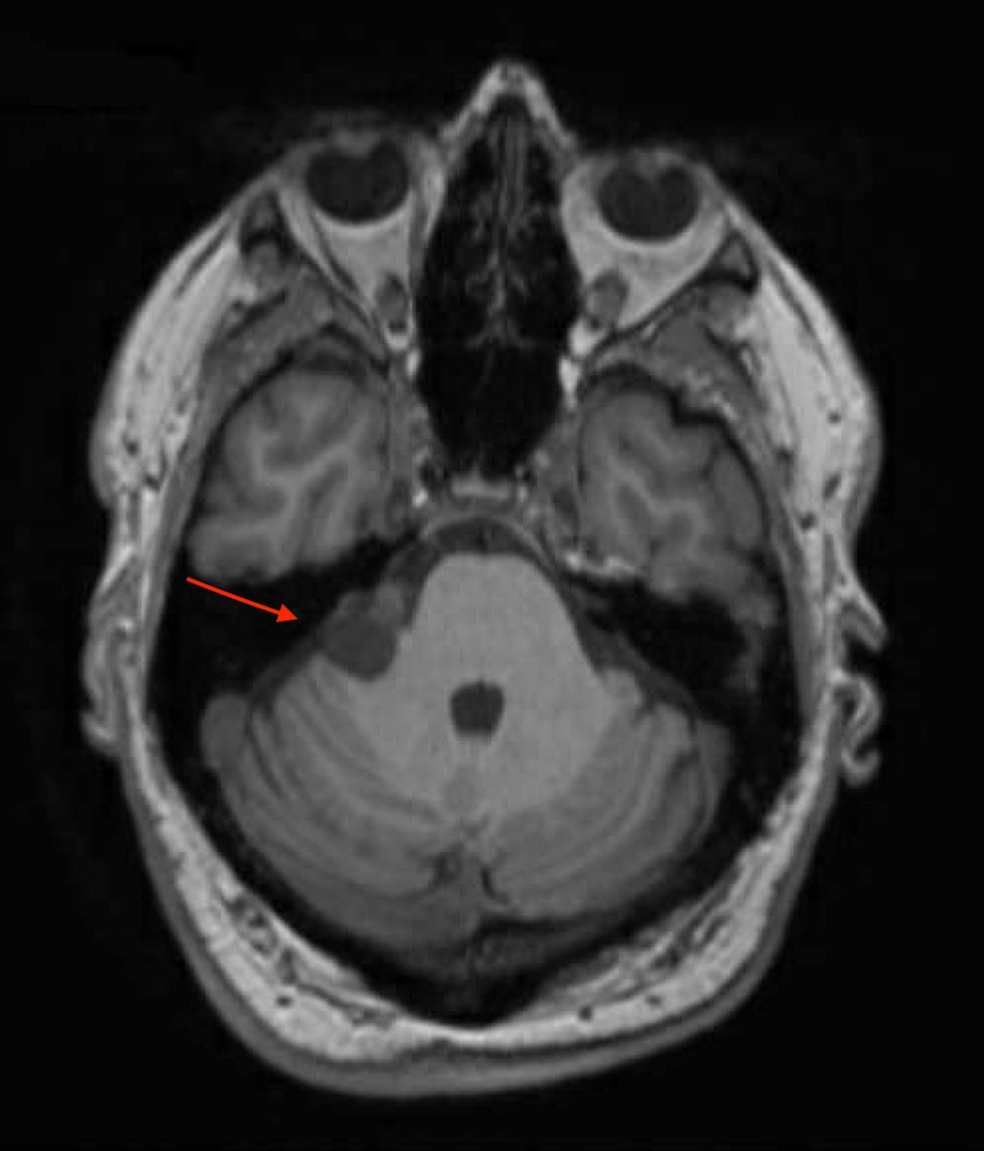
Preoperative T1-weighted MRI with contrast demonstrating right CPA mass indicated by the red arrow CPA, cerebellopontine angle
